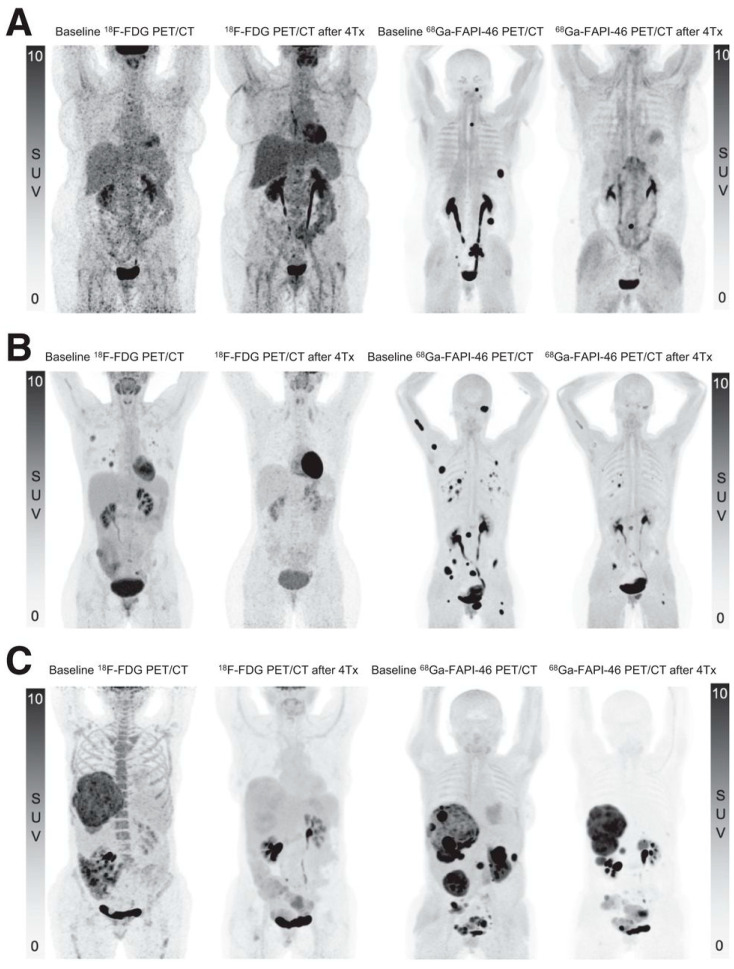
Near-complete metabolic response in three patients with solitary fibrous tumors (SFT). Baseline and follow-up PET/CT MIP images ([^18^F]FDG and [^68^Ga]Ga-FAPI-46) after four cycles of [^90^Y]Y-FAPI-46 in three patients with SFT. (A) A 58-year-old woman with primary retroperitoneal SFT, progressive after conventional treatments with lymph node and soft tissue metastases. (B) A 38-year-old woman with lung, lymph node, peritoneal and bone metastases. (C) A 61-year-old woman with liver and peritoneal metastases. Figure reproduced from Lanzafame et al., ^90^Y-FAPI-46 Theranostics Leads to Near-Complete Metabolic Response in 3 Patients with Solitary Fibrous Tumors. J Nucl Med. 2025 Sep 2; doi: 10.2967/jnumed.125.269572 [54]. under CC BY 4.0 (https://creativecommons.org/licenses/by/4.0/, accessed on 3 September 2025).
